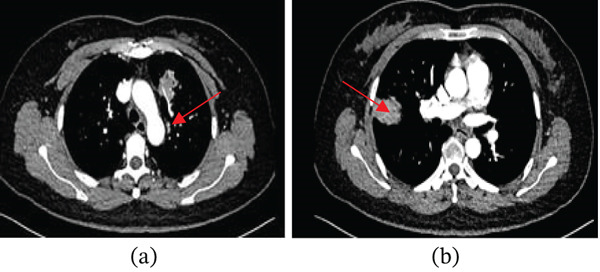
(a) Enhanced chest CT demonstrates significant enhancement of the lesion in the lingular segment of the left upper lobe, with visible vascular shadows within the lesion (arrow). (b) Enhanced chest CT demonstrates significant enhancement of the lesion in the right upper lobe.
